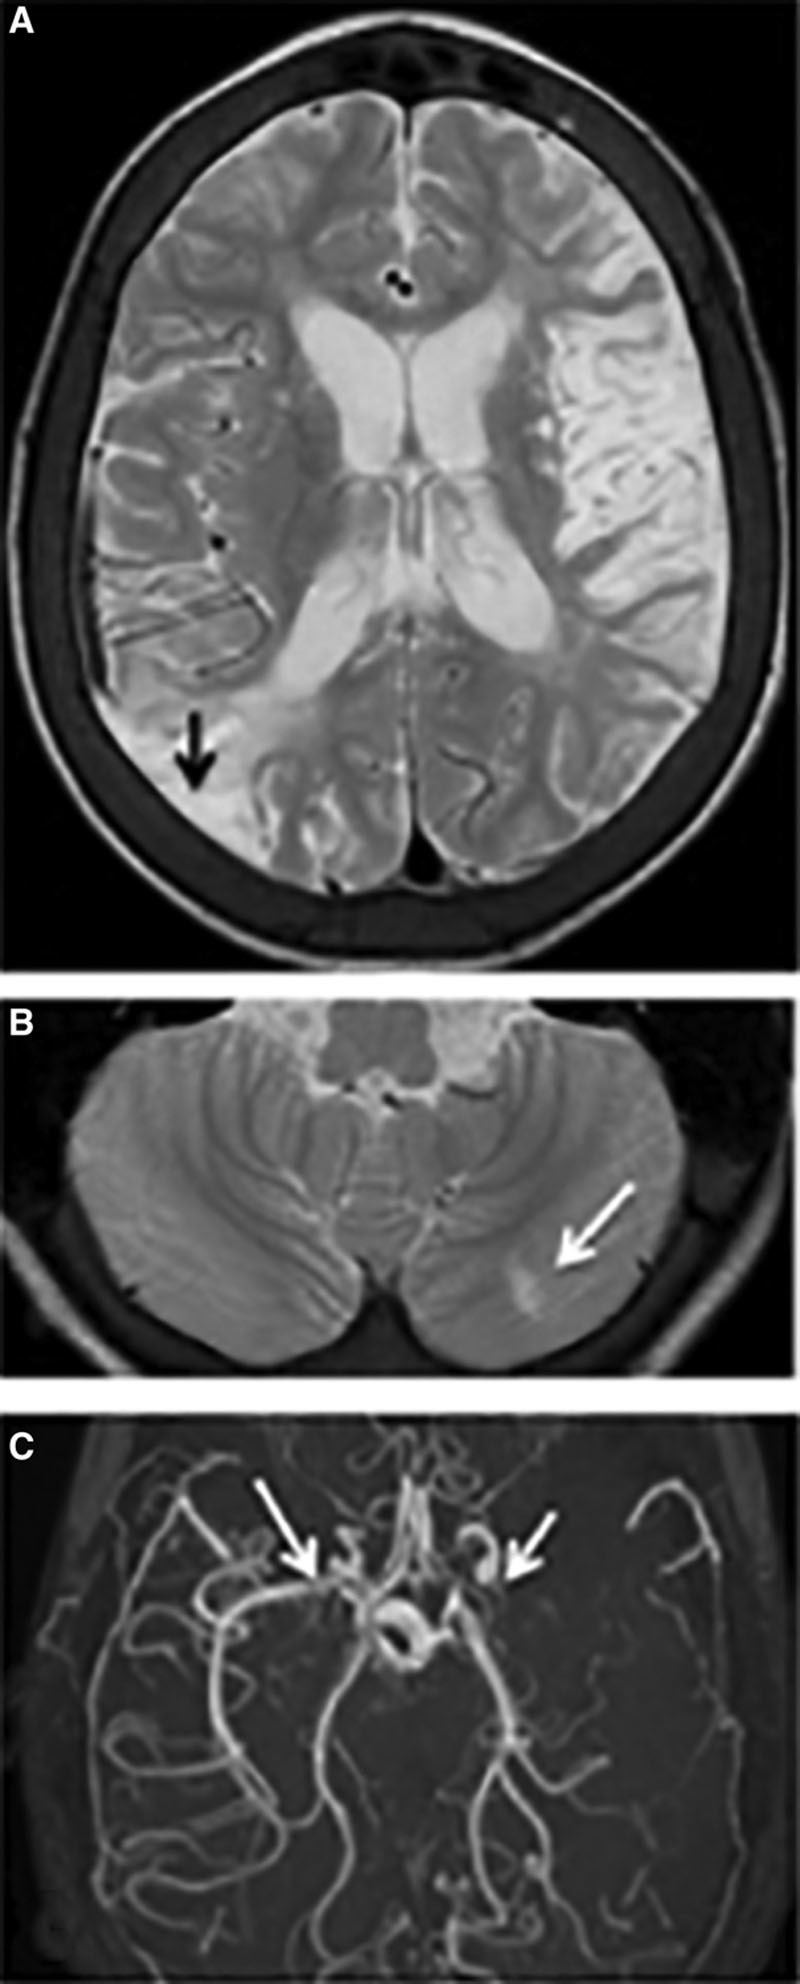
Ten-y-old Tanzanian girl with seizures and focal weakness with absent transcranial Doppler velocities. A, Axial T2-weighted magnetic resonance image showing mature right MCA (middle cerebral artery)/PCA (posterior cerebral artery) watershed territory (black arrow) and left MCA territory infarcts. Bilateral deep gray and deep white matter watershed lesions are seen bilaterally. B, A small left cerebellar infarct (white arrow) is seen in the same patient. C, The magnetic resonance angiogram reveals an occluded left MCA (short arrow) and a narrow right MCA (long arrow). The PCAs appear normal. Marrow expansion of the skull vault is noted. The patient subsequently died.
